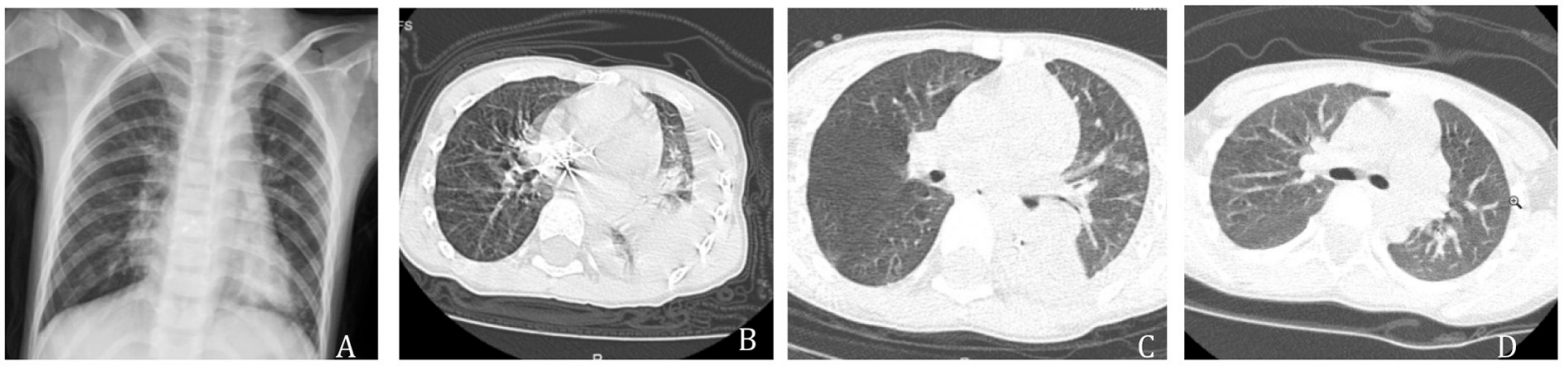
(A) Pre-admission outpatient chest X-ray showed inflammation in both lungs. (B) On admission, chest CT showed inflammation in both lungs, partial atelectasis in the left lower lobe, and left pleural effusion. (C) Three days after closed chest drainage, a chest CT showed localized progression of inflammation in both lungs, partial atelectasis in the left lower lobe, and resorption of left pleural effusion. (D) Eighteen days after antibiotic treatment, chest CT showed improvement of the inflammation in both lungs, and a small amount of pleural effusion on the left side.
